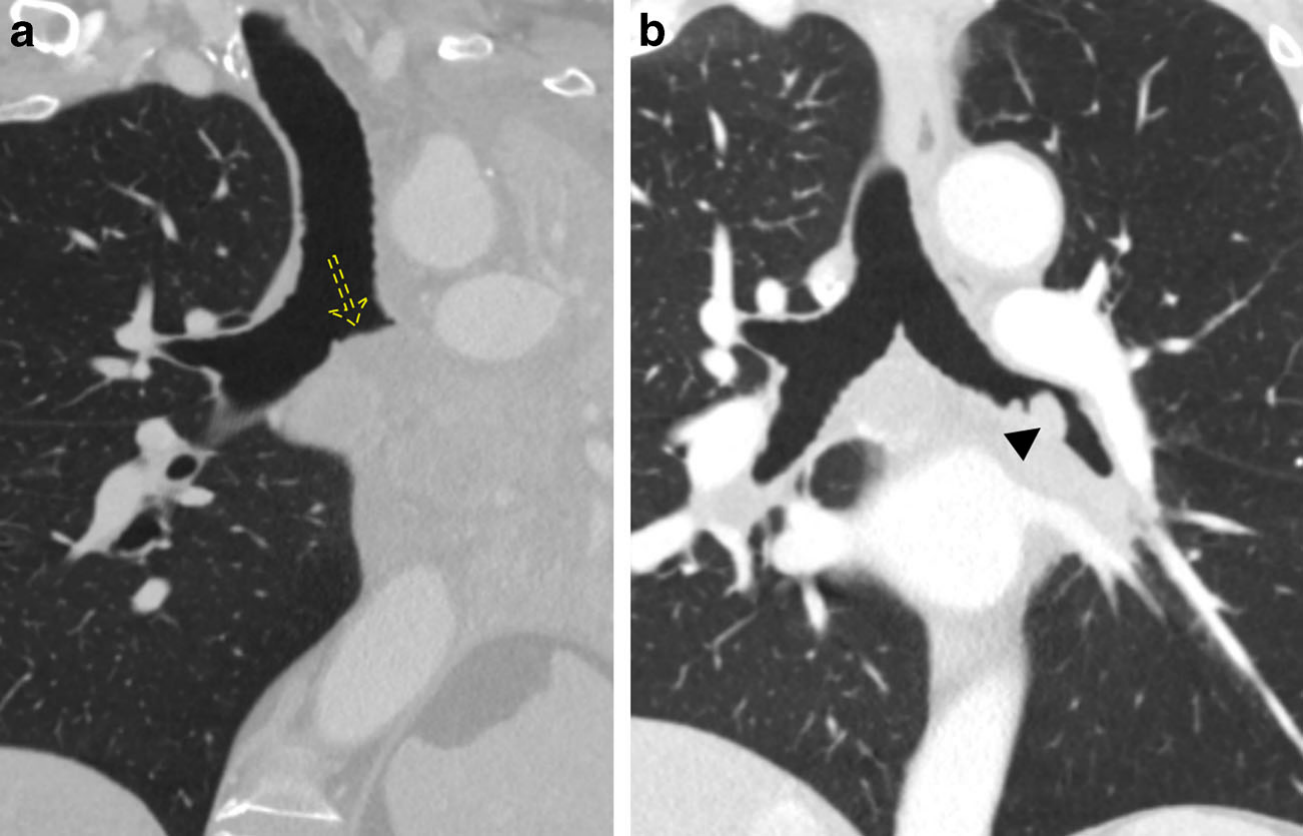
Bronchial obstruction due to neoplasm. Coronal CT imaging of metastatic renal carcinoma before (a) and after (b) chemotherapy. Note that prior to chemotherapy (a) there is obstruction of the left main bronchus with an air fluid level (*dashed arrow*) and complete collapse of the left lung. After several rounds of chemotherapy (b) the endoluminal metastatic renal cell carcinoma is now seen (*arrowhead*) and is no longer obstructive with interval re-expansion of the left lung
